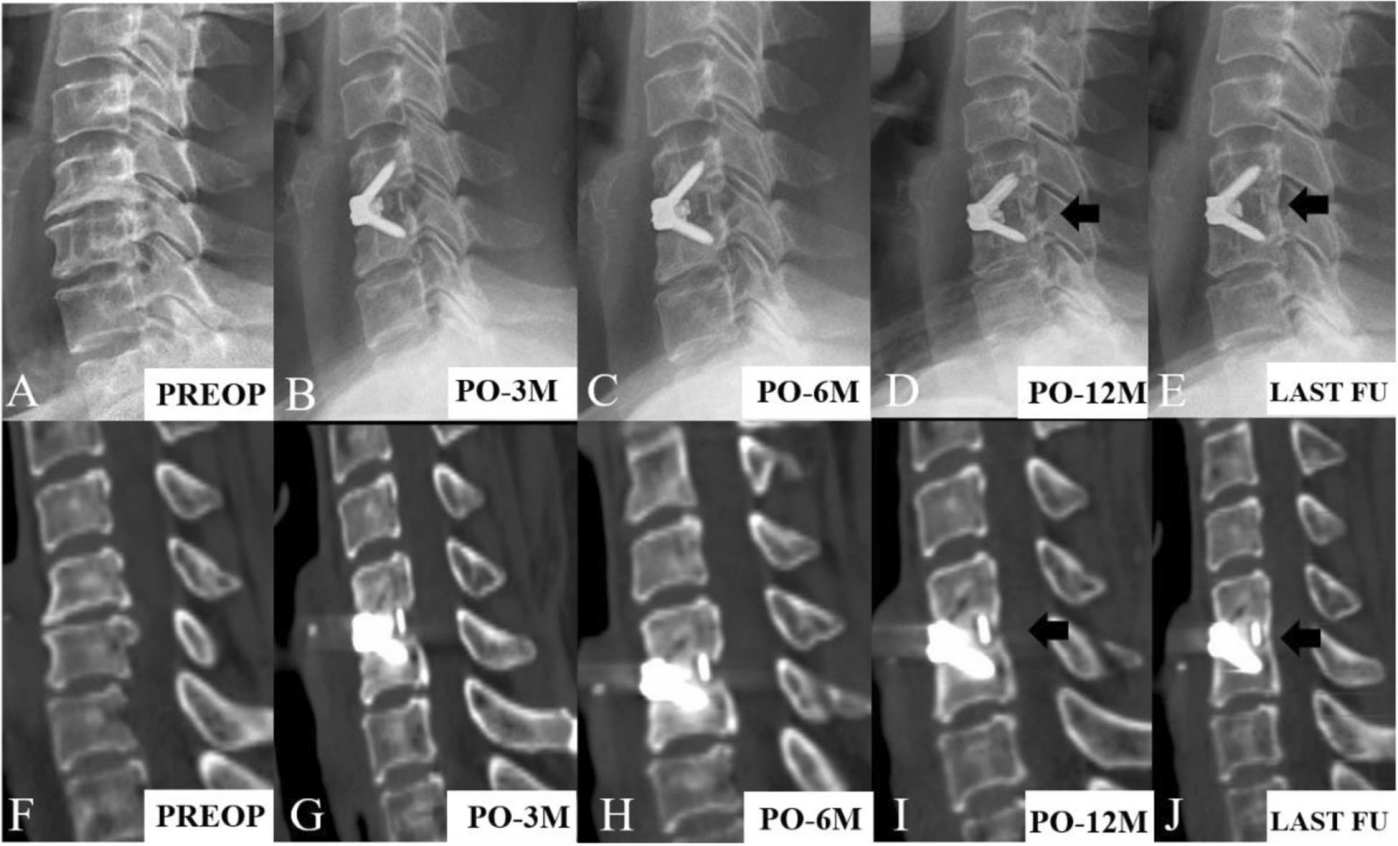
Assessment of fusion status in the lateral radiographs and CT scan images. The bridging bone was observed at the surgical level (black arrow)
